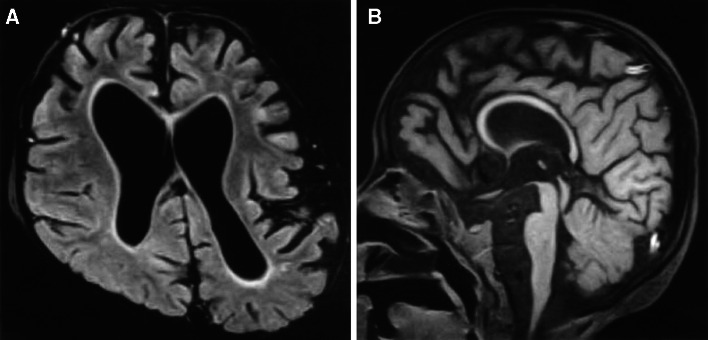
a Magnetic resonance imaging (MRI), T_1_-weighted, 1.5T axial view (*SE* spin echo; TR = 15 ms; TE = 450 ms). MRI reveals dilatations of lateral ventricles and bifurcated septum pellucid. b The splenium of the corpus callosum is thin
